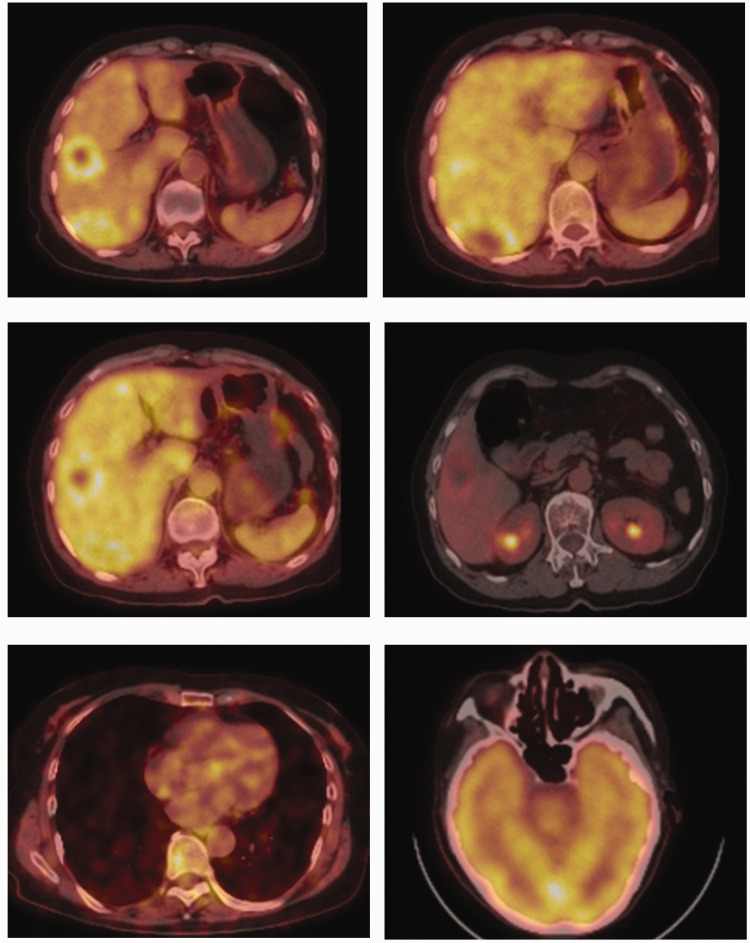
Positron emission tomography-computed tomography shows multiple intrahepatic space-occupying lesions and increased glucose metabolism. No increased glucose metabolism was observed in the lungs or skull.
